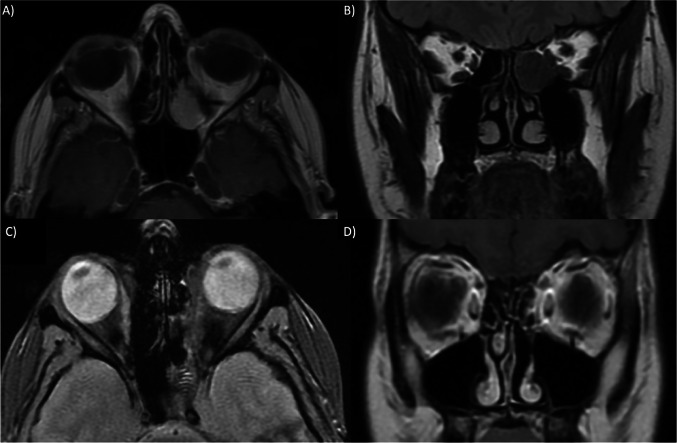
Preoperative imaging A, B: A MRI in T1 showing an ethmoidal mass in axial view; B Same MRI but in coronal view showing a mass attached to the lamina papyracea. Postoperative 6 months follow-up imaging C, D: C MRI in T2 showing surgical results in axial view; D Same MRI but in coronal view showing the absence of ethmoidal mass
